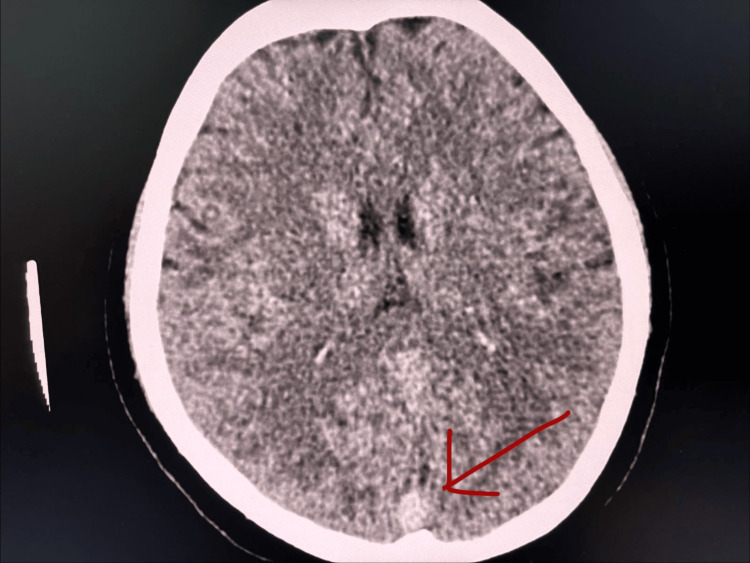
"Dense" delta sign in non-contrast CT brain
